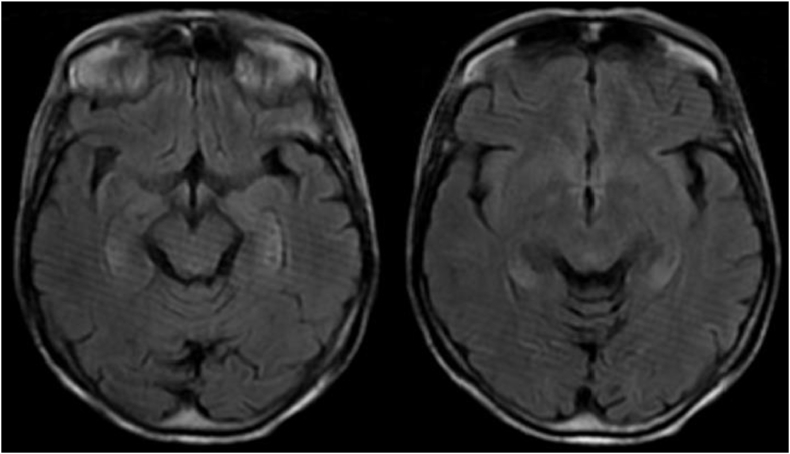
Axial T2-weighted FLAIR imaging demonstrate bilateral temporal hyper intensity.
